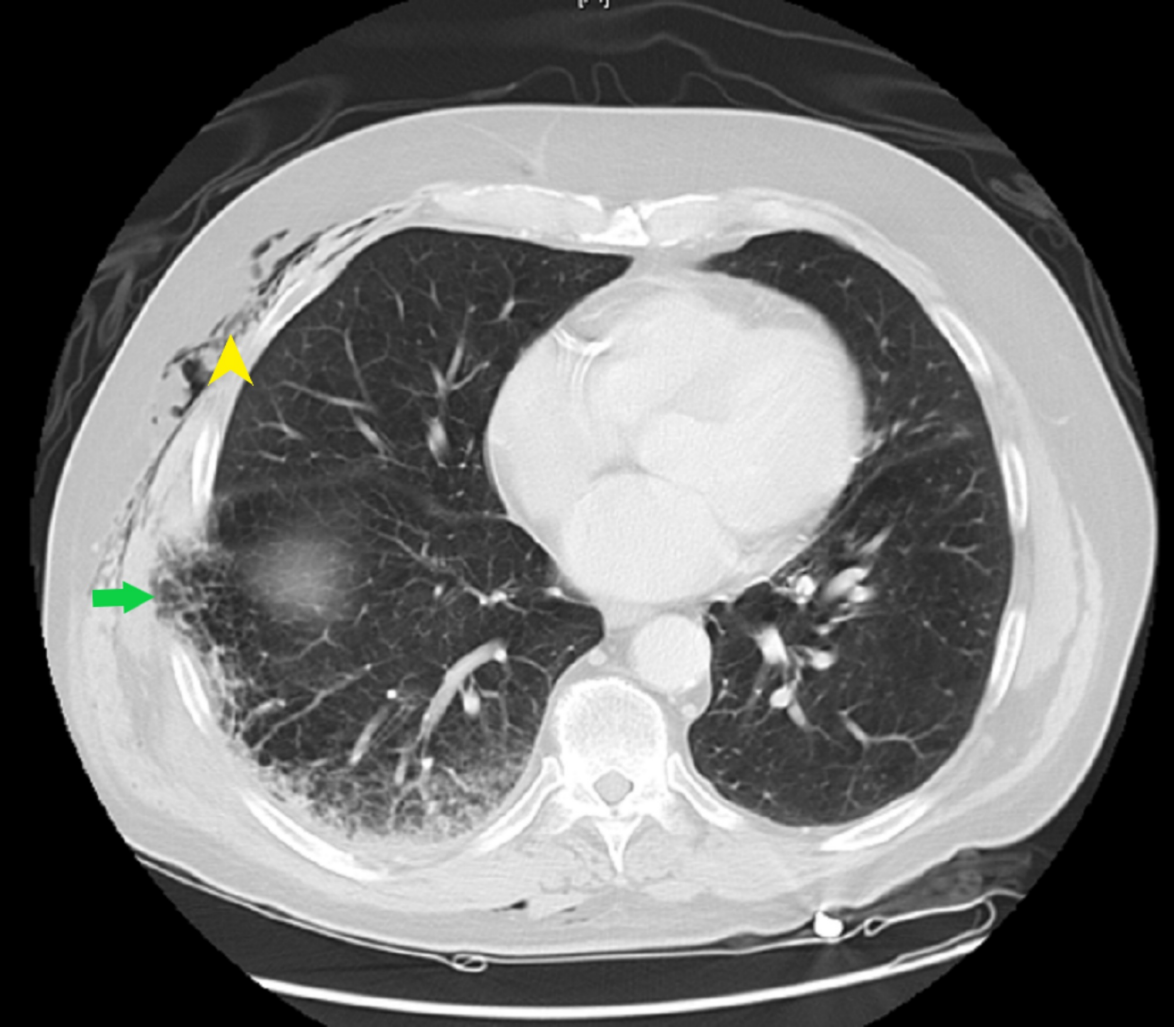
CT chest axial view showing a small portion of the right lung herniating through the right posterolateral seventh and eighth rib space (arrow) with mild subcutaneous emphysema (arrowhead) CT: computed tomography
